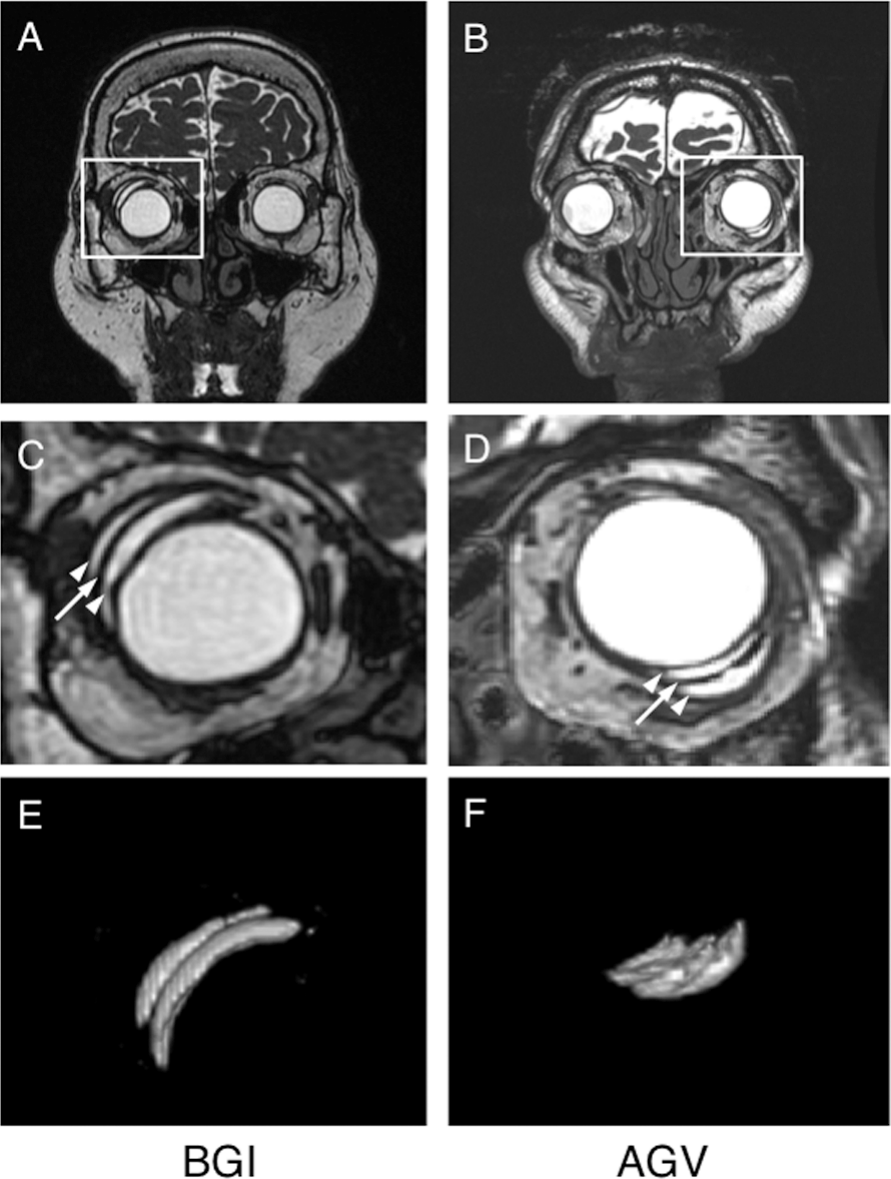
Magnetic resonance imaging (MRI) of the orbit. Coronal images show a Baerveldt Glaucoma Implant (BGI) in the superotemporal quadrant (A, C) and an Ahmed Glaucoma Valve (AGV) in the inferotemporal quadrant (B, D) 6 months postoperatively. With high magnification views (C, D), layers of fluid accumulation with the same intensity as intraocular fluid (arrowheads) are seen under (inner bleb) and over (outer bleb) the low-intensity area that corresponds to the endplate (arrows). With three-dimensional views of filtering blebs obtained by the volume-rendering process (E, F), the inner and outer blebs are seen clearly. In these cases, the bleb volume is calculated to be 646.0 mm^3^ after BGI (E) and 272.2 mm^3^ after AGV implantation (F).
